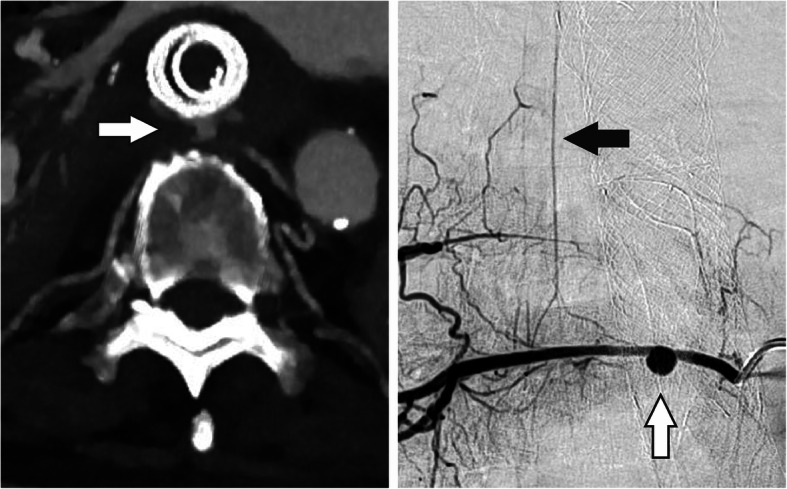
DSA and CT scan before implantation of the *PK Papyrus Stent. *In the CT scan the pseudoaneurysm can be detected ventral of the spine with direct contact to the esophagus. Furthermore, the previously implanted, esophageal stents can be seen. The esophageal tissue seems to be thinned out. After the CT scan, the DSA confirms the suspected pseudoaneurysm and shows the connection between the intercostal artery and the anterior spinal artery. White arrow: Pseudoaneurysm of the intercostal artery. Black arrow: Anterior spinal artery
